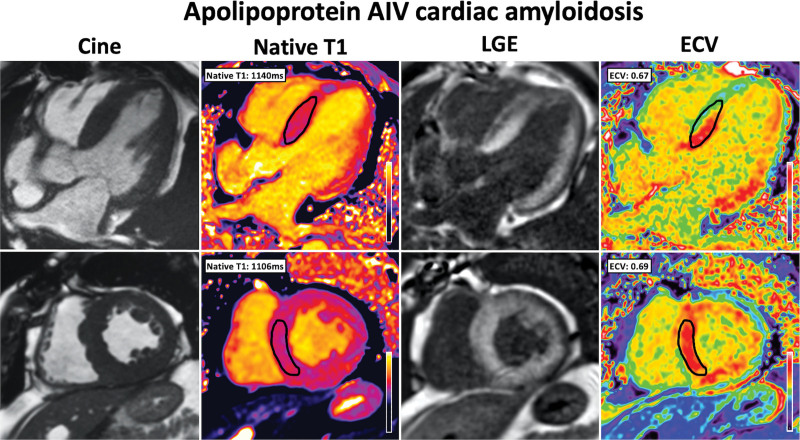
Cardiac magnetic resonance images in Apo AIV cardiac amyloidosis demonstrate classical features of cardiac amyloidosis. Cine images were acquired with steady-state free precession (SSFP) sequence, and demonstrate increased wall thickness and left ventricular mass. Native-T1 mapping was acquired using the modified look-locker inversion (MOLLI) recovery sequence and demonstrated an elevated native-T1. Late gadolinium enhancement (LGE) imaging was acquired using phase-sensitive inversion recovery sequence reconstructions with SSFP read-outs and demonstrated diffuse biventricular transmural LGE. T1 mapping was repeated 15 minutes postcontrast using the same slice locations with the MOLLI sequence to produce automated inline extracellular volume (ECV) mapping reconstruction and demonstrated an elevated ECV.
